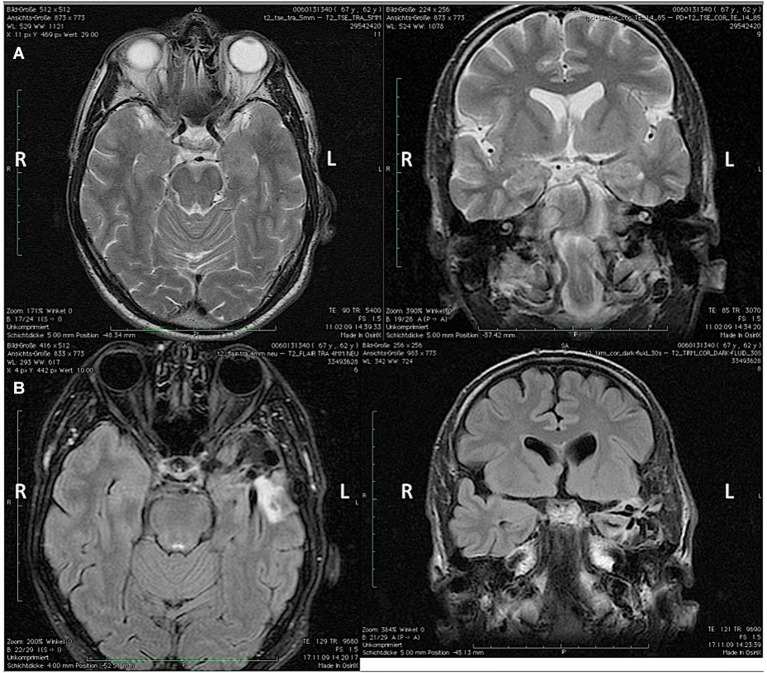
(A) Pre-surgery axial (left) and coronal (right) MRI and (B) post-surgery coronal (left) and axial (right) MRI showing the apical temporal lobe resection and the shrinkage of the hippocampal remnant. L = Left hemisphere; R = Right hemisphere.
